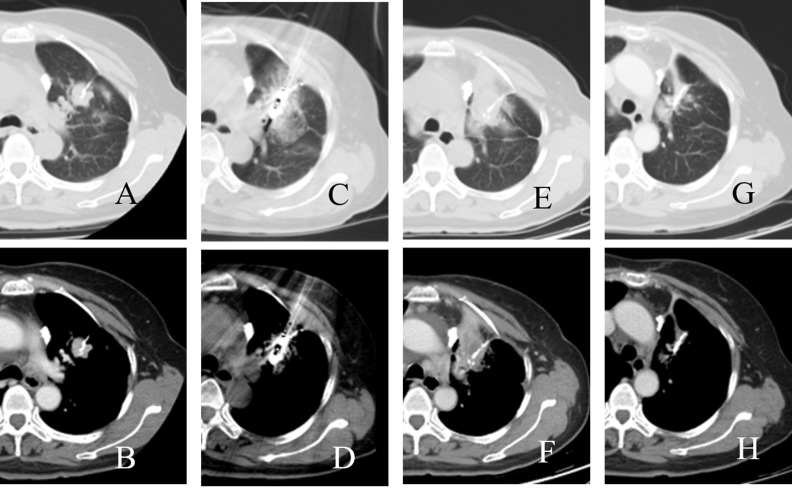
CT images of a patient who underwent microwave ablation A., B. before microwave ablation; C., D. during microwave ablation E., F.; three months after microwave ablation; G., H. 54 months after microwave ablation.
